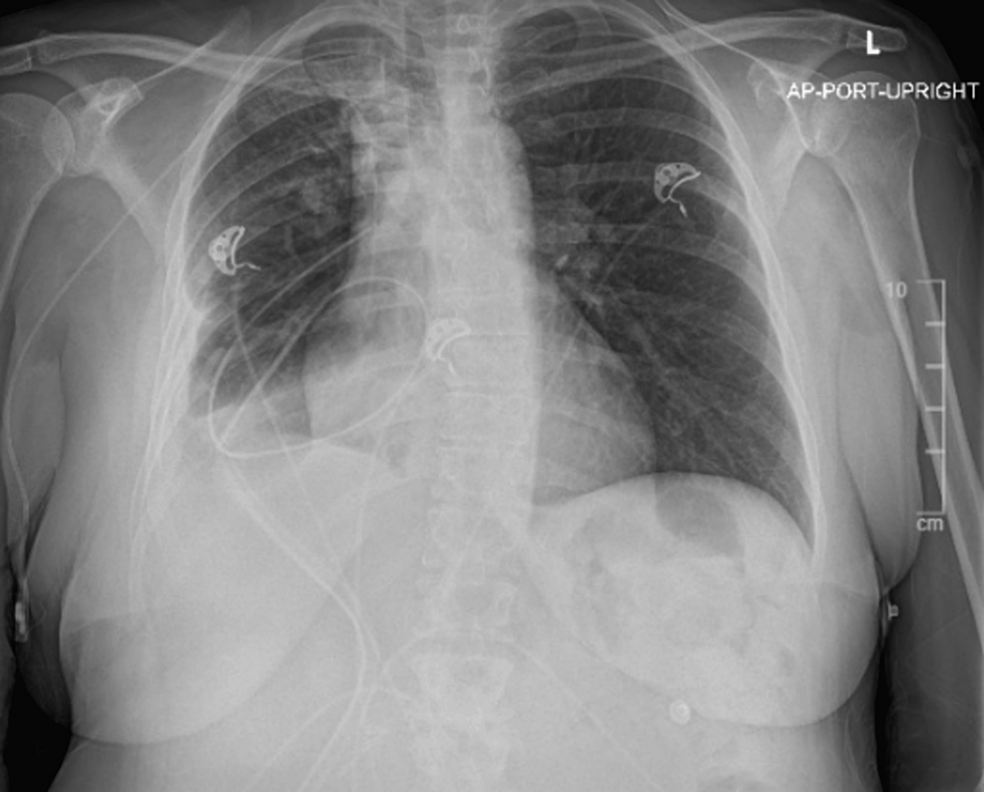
Final Chest X-ray Before Discharge
